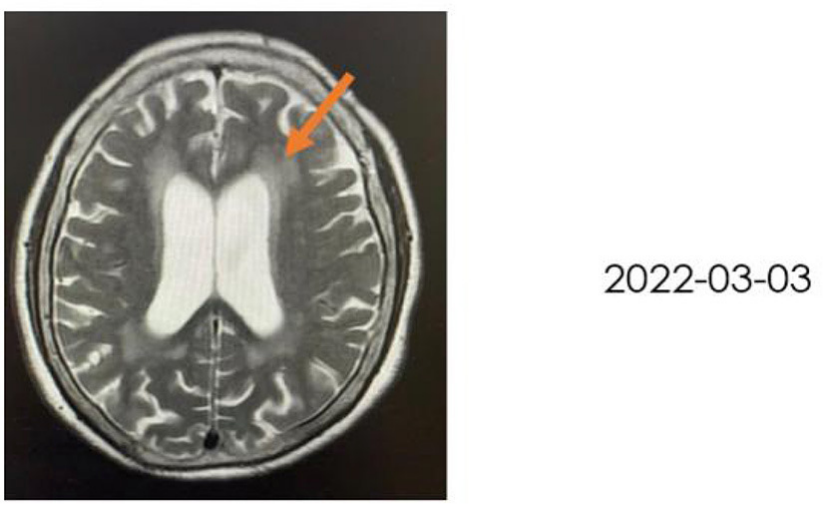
Brain MRI took on 3 March 2022, revealing significantly reduced ventricles.
